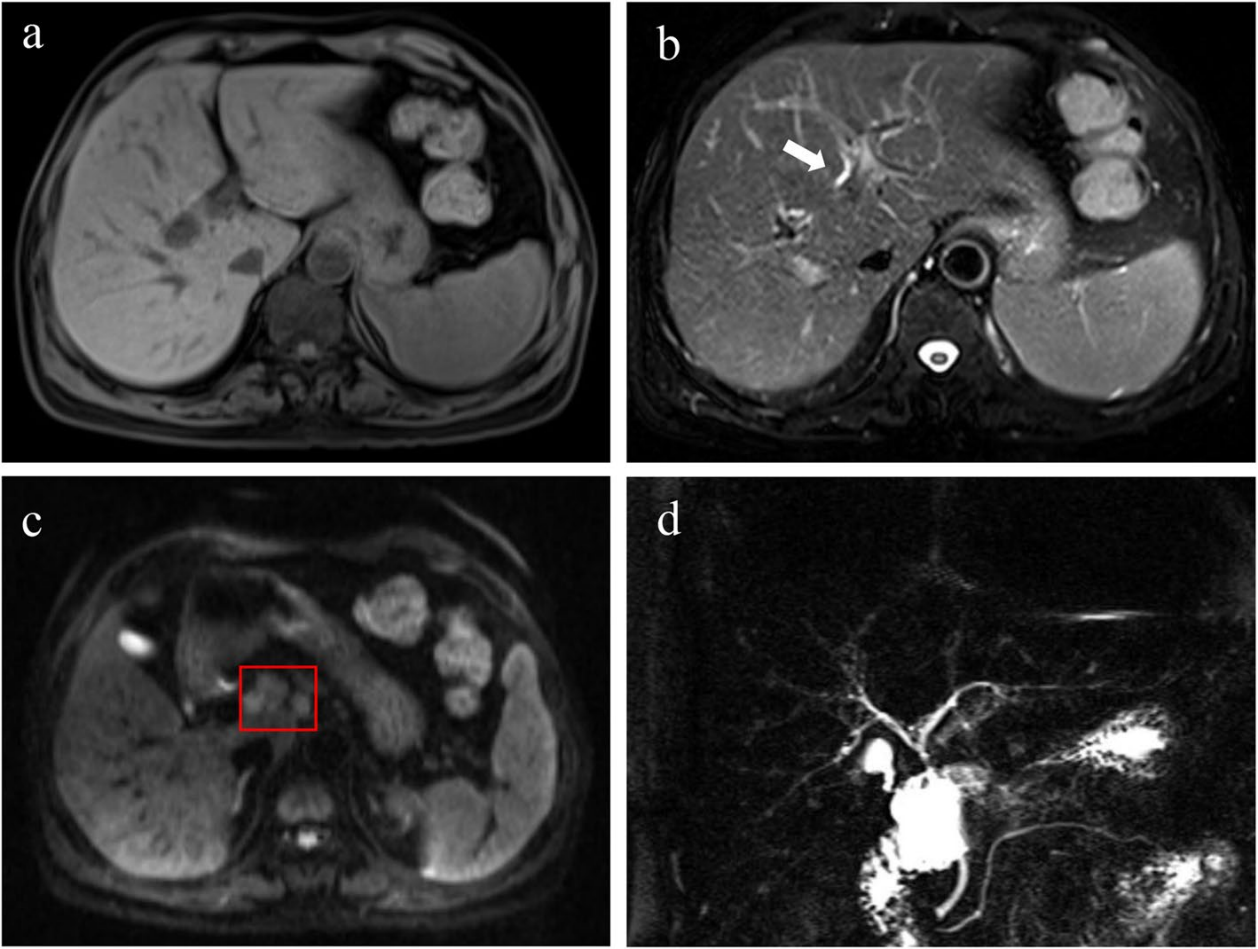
Non-enhanced axial MRI scans in a 52-year-old female with PBC (stage II–III, Ludwig staging system) (a–d). The T1WI image shows the liver with a regular contour and normal distribution of lobes (a). The T2WI image shows periportal hyperintensity (arrows), and mild heterogeneity of liver parenchyma (b). The diffusion-weighted image (*b* = 500 s/mm.^2^) shows lymphadenopathy (hepatic hilar, red box) (c), and the MRCP image shows irregular morphology, segmental stenosis, and dilatation of the intrahepatic medium-small bile ducts (d)
